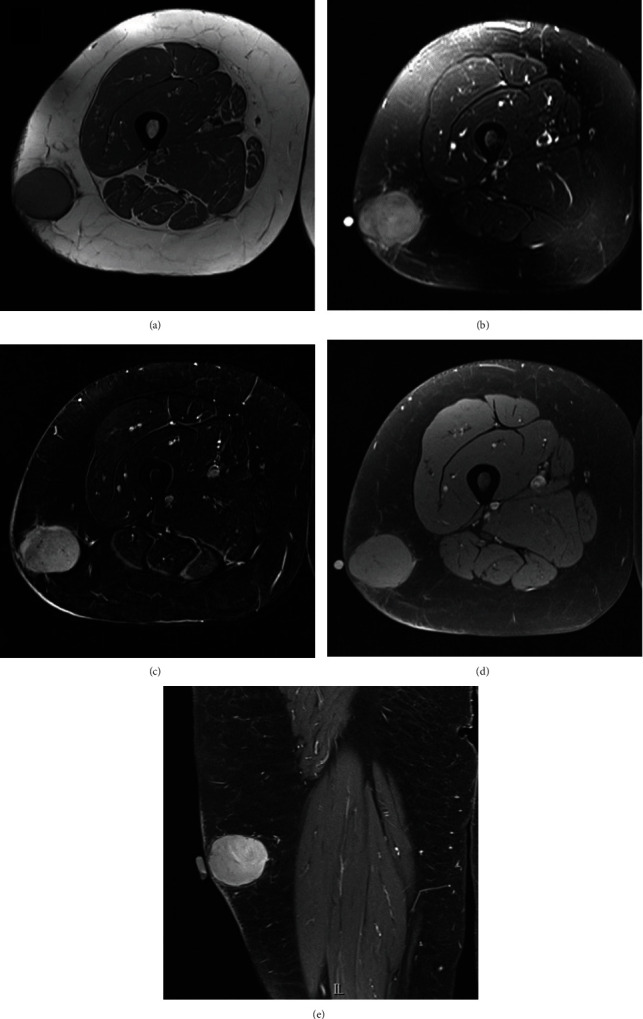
MRI (a) axial T1 WI nonfat saturated demonstrates mild hyperintensity compared to skeletal muscles. (b) Axial T2 WI with fat saturation demonstrates marked homogenous hyperintensity. (c) Axial subtraction image (pre- and post-IV gadolinium contrast) T1 WI with fat saturation confirms the homogeneous avid enhancement. (d) Axial T1 WI with fat saturation, precontrast demonstrates mild hyperintensity compared to skeletal muscles. No fat signal suppression. (e) Coronal T1 WI with fat saturation, post-IV gadolinium contrast injection demonstrates homogeneous avid enhancement.
